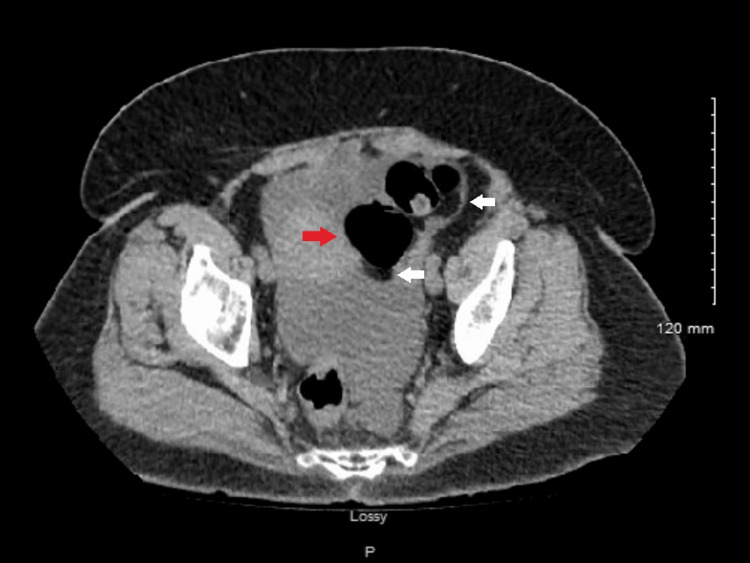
CT angio abdomen and pelvis (axial view) White arrows demonstrate wall thickening with edema along the proximal and mid small bowel. The red arrow demonstrates increased enhancement along the small bowel loop.
